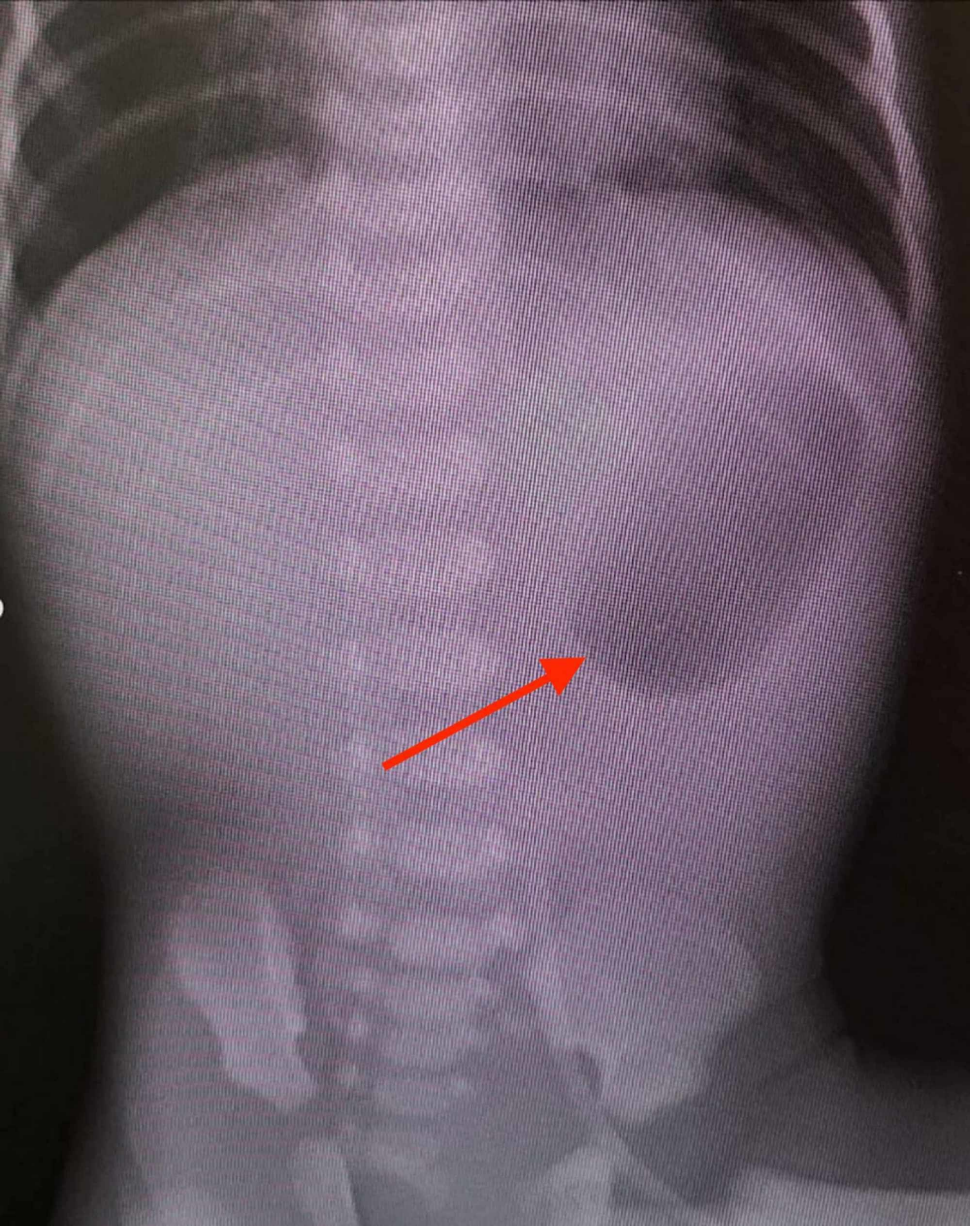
An abdominal radiograph demonstrating a single bubble sign (red arrow), which aligns with the diagnosis of pyloric atresia
